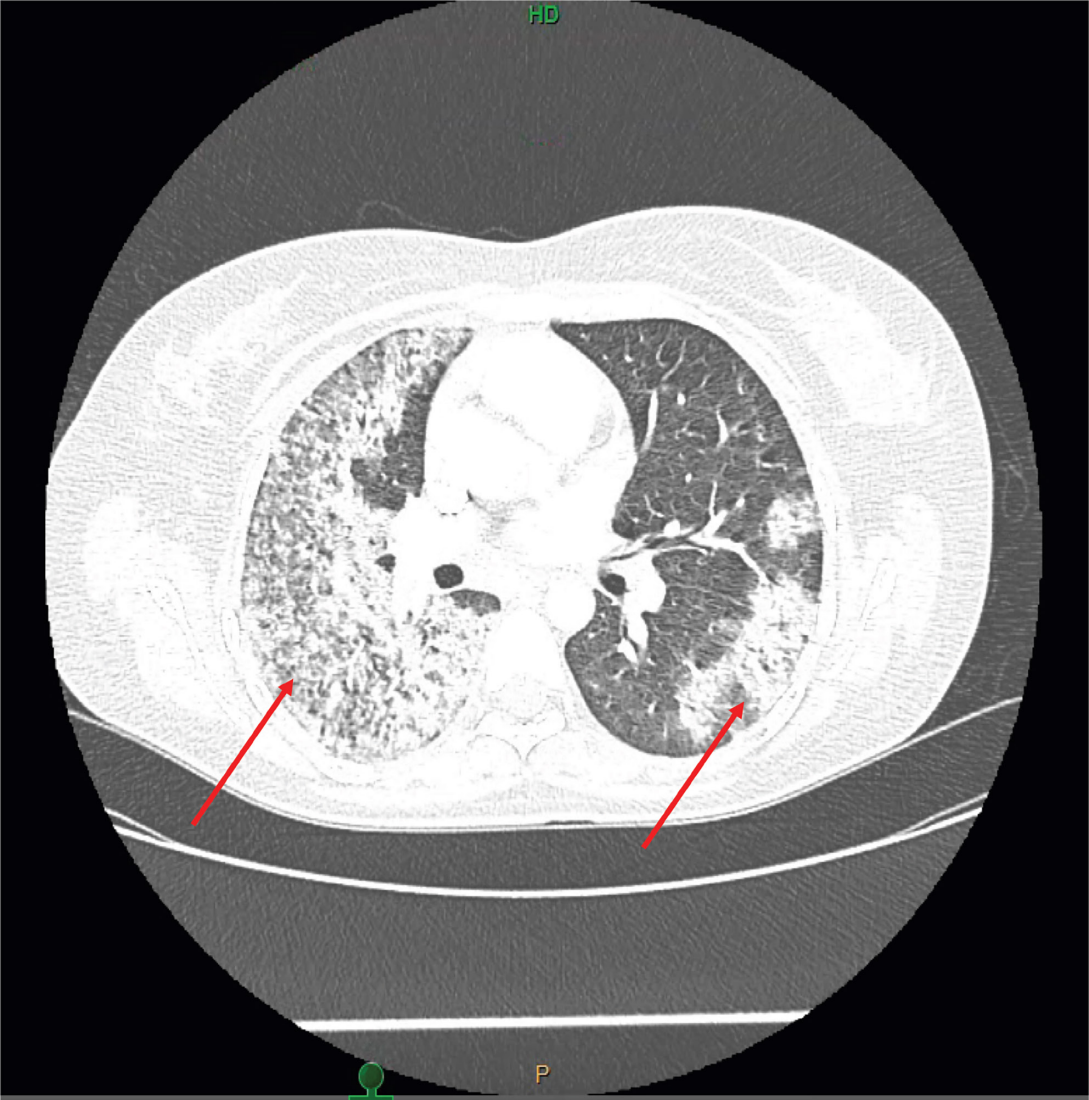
Computed tomography (CT) chest showing dense consolidation of the entire right lung and peripheral infiltrates in the left lung.
